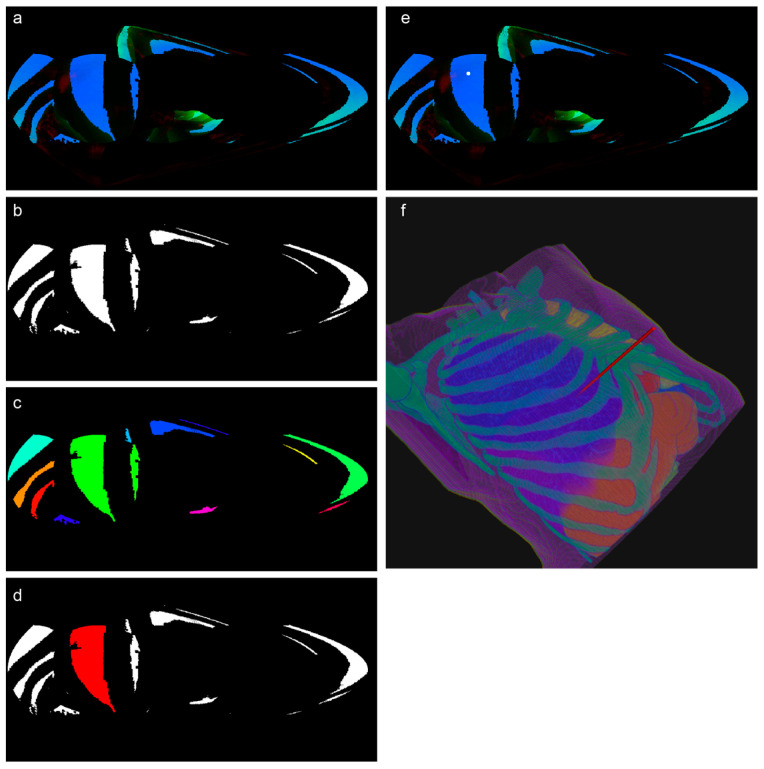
From illumination map to puncture path: visual biopsy trajectory planning. (a) RGB Sinusoidal Projection: Illumination results (attenuation terms and obstacle indicator) mapped to RGB channels and projected onto a 2D planar image using sinusoidal projection, visualizing feasible puncture regions while preserving area ratios. In the RGB projection, the blue, red, and green channels, respectively, represent the intensity parameters for ‘ref’, ‘vas’, and ‘trans’ terms. (b) Initial Region Segmentation: Low-risk regions are segmented by applying statistical thresholds to the RGB values, determining the RGB value ranges for safe regions based on the statistical distribution of the RGB channels, thus identifying potential areas of interest. (c) Filtered Safe Regions: Filtered safe regions were obtained after setting a size threshold based on clinical experience to remove smaller candidate regions. (d) Expert Annotation: Expert annotation by a thoracic surgeon who selected a recommended needle insertion region based on their clinical experience. (e) Final Puncture Point: Final puncture point determined by expert annotation or machine learning prediction and refined to a single pixel via iterative erosion (indicated by a white dot). (f) Biopsy Trajectory: 2D puncture point reverse-mapped to 3D CT space, visualizing the calculated biopsy needle trajectory (red cylinder).
